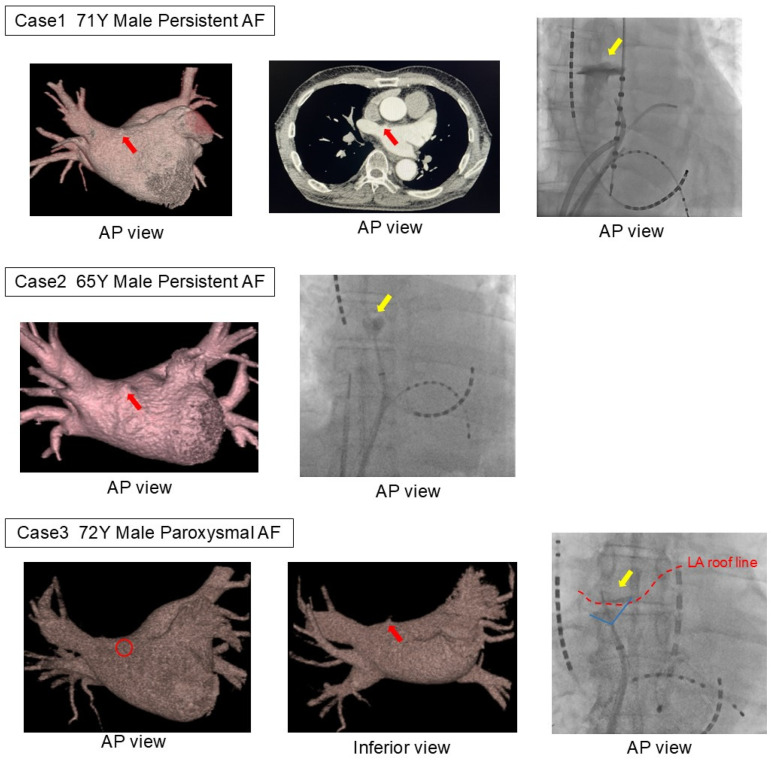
Three patients with LAD-related complications. In all three cases, extravasation was observed during pulmonary vein angiography using an angioburman catheter. In all cases, an LAD was found on the anterior side of the right superior pulmonary vein, the common site of the LAD. The catheter had inadvertently entered the diverticulum during contrast injection immediately after transitioning from angiography of the left pulmonary vein to that of the right pulmonary vein. The red arrow indicates the left atrial diverticulum on the CT image, the yellow arrow points to the extravasation of contrast observed in the fluoroscopic image, and the red dashed line represents the roof line of the left atrium. LA, left atrium; LAD, left atrial diverticulum.
